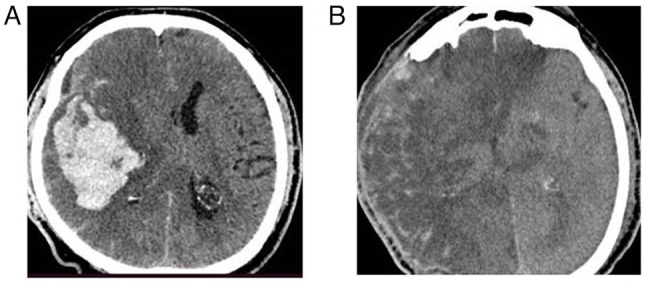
Fronto-temporoparietal craniectomy in a patient with other pathologies (female patient, 64 years of age, who presented with subarachnoid hemorrhage and intracerebral hemorrhage after anterior communicant artery aneurysm rupture and who underwent a right-sided craniectomy with a poor outcome and left hemiplegia). (A) Pre-operative CT scan, axial view; (B) post-operative CT scan, axial view.
